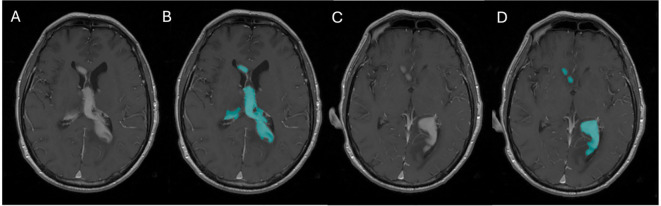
Example of manual segmentation using ITK-SNAP software. Post-contrast axial T1-weighted MR images from a single patient with intra- and periventricular PCNSL, at two cross-sectional levels (A, C). Corresponding segmentations (B, D) display cyan overlays indicating manually segmented enhancing tumour volumes used for volumetric analysis.
